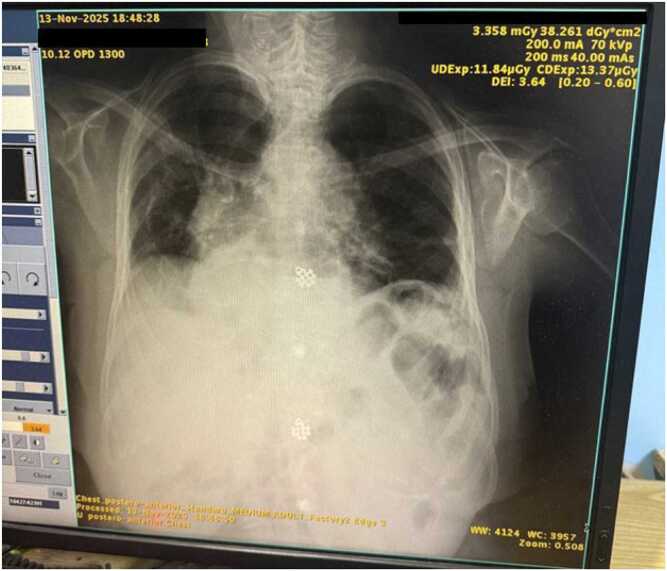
Follow-up chest X-ray (13 November 2025): marked radiological improvement with resolution of consolidations.
